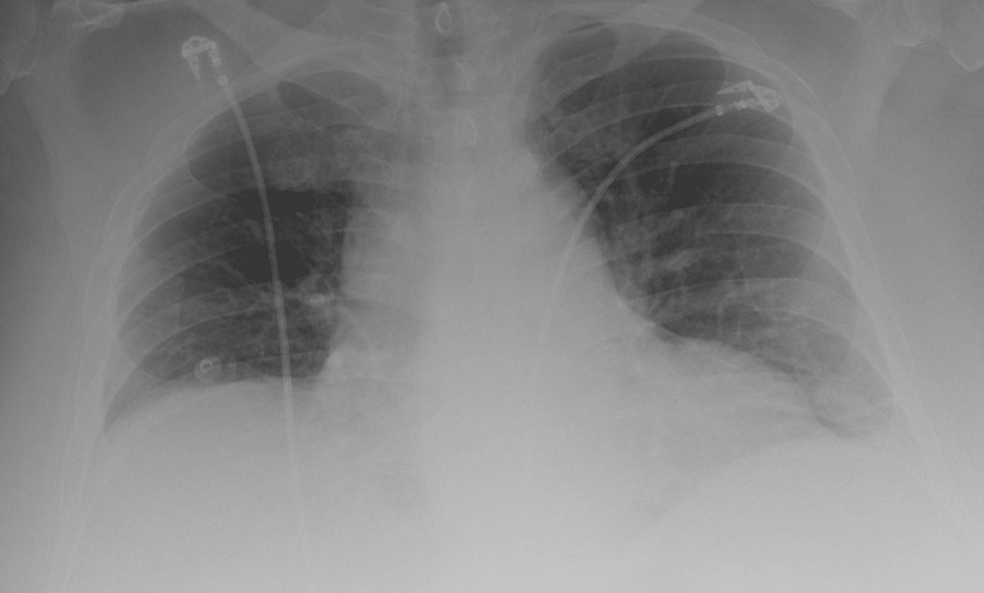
Chest X-ray on presentation revealing bilateral infiltrates suggestive of viral pneumonia with some bibasilar pleural effusions.
